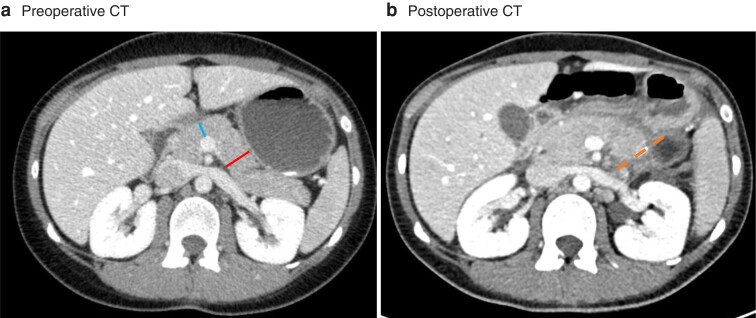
Pancreatic thickness measurements a Preoperative and b postoperative CT images. a Pancreatic thickness was measured at the transection site (23 mm, right [red] line), and at the neck of the pancreas (12 mm, left [blue] line). Main pancreatic duct diameter was measured at the same locations (not marked in this figure), and 1 mm was entered if not visible. The dashed (orange) line in b represents the transection site (body of pancreas in this patient).
